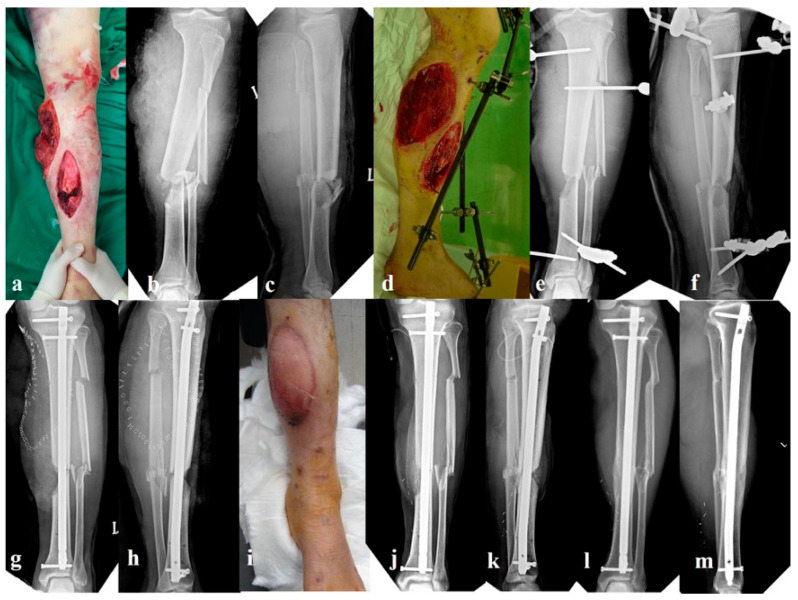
A 35-year-old man sustained a Gustilo–Anderson type IIIB open tibial shaft fracture (Arbeitsgemeinschaft für Osteosynthesefragen/Orthopaedic Trauma Association 42-B) following a traffic accident. Initial photographs and radiographs show a comminuted open fracture with extensive soft-tissue loss (a–c). After thorough irrigation and debridement, provisional stabilization was achieved using a uniplanar external fixator without application of a reduction miniplate (d–f). Definitive fixation with intramedullary nailing and rotational flap coverage was performed in the staged setting (g,h). After flap healing, autogenous bone grafting was performed to address the cortical defect (i–k). Final follow-up radiographs show solid bone union with satisfactory alignment and no signs of infection (l,m).
